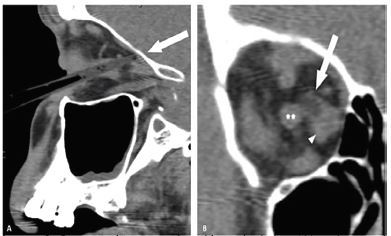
Computed tomography with sagittal view (A) and coronal view (B) showing the foreign body (arrow) entering the orbit, adjacent to the right medial rectus muscle (arrowhead) and abutting the optic nerve sheath (white asterisks in middle of figure) and terminating at the posterior superior orbital wall with no evidence of orbital wall fracture.
